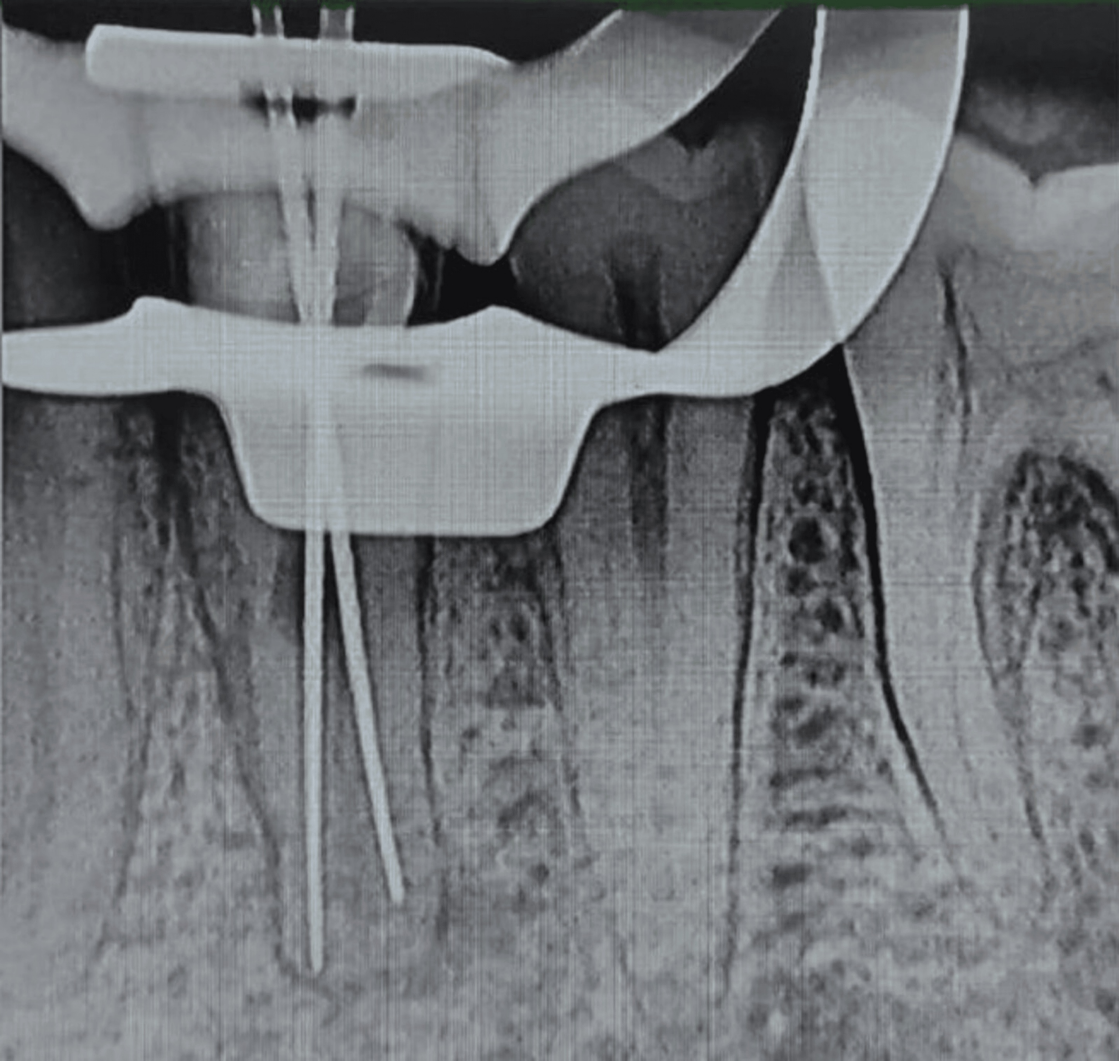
Biomechanical preparation was done using hand-operated files and nickel-titanium rotary instruments (Dentsply Maillefer, Ballaigues, Switzerland). Thorough irrigation with 5.25% NaOCl, normal saline, and 0.2% chlorhexidine was also done simultaneously. The master cone fit was checked and confirmed on the radiograph following the final working length determination
